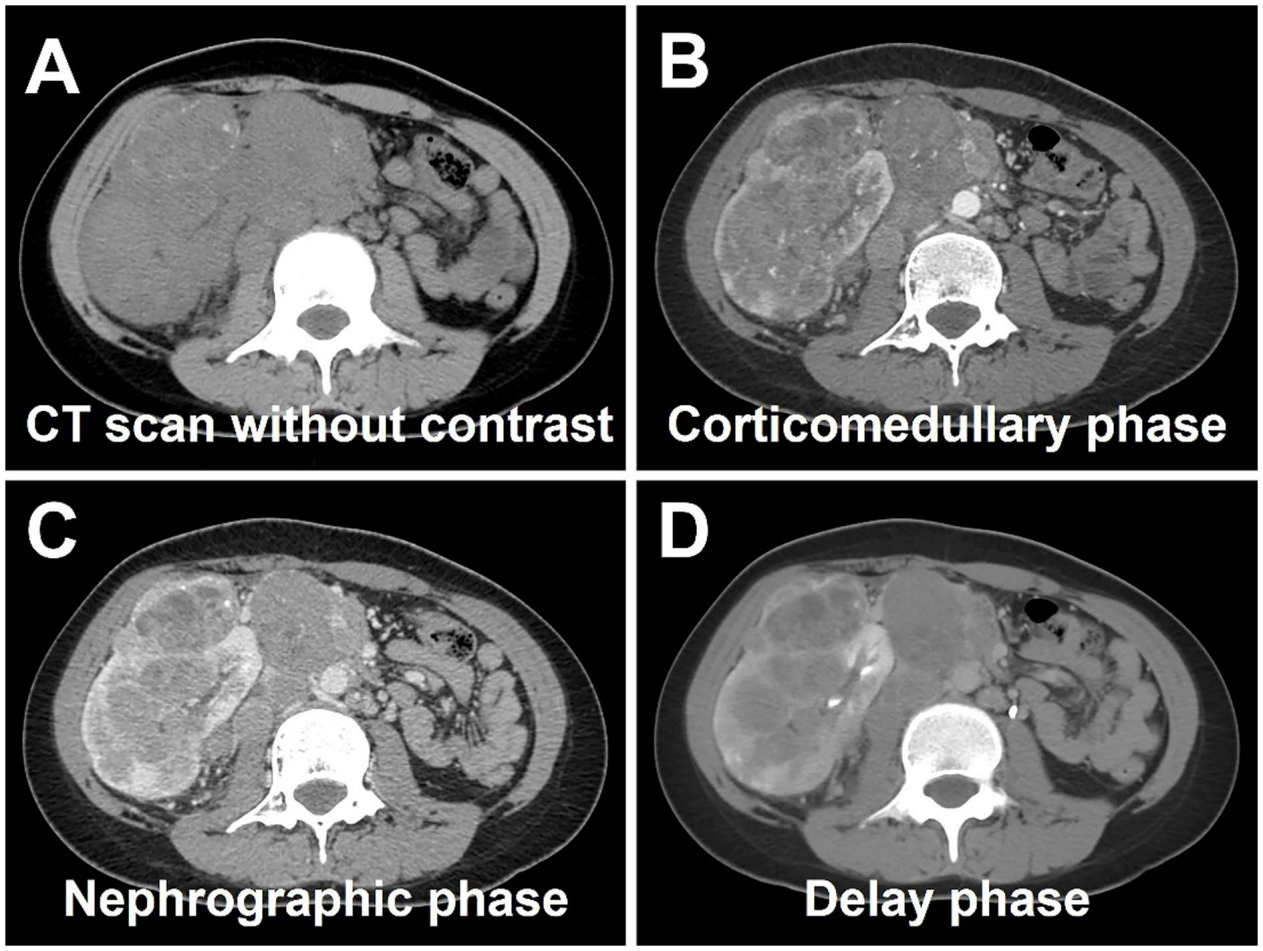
Computed tomography (CT) images of Xp11.2 RCC. (A) The transverse image shows a right renal mass measuring approximately 5.2 cm × 7.1 cm × 8.8 cm with multiple calcifications. (B–D) The CT attenuation values of corticomedullary phase, nephrographic phase, and delayed phases were 83 HU, 82 HU, and 75 HU, respectively. The solid component of the mass displayed heterogeneous marked enhancement, with areas of hypodense necrosis observed within. Several fused lymph nodes were identified in the medial right kidney and retroperitoneum, showing the same density and enhancement pattern as the right intrarenal lesion.
